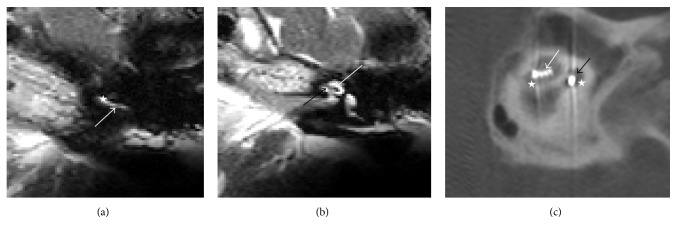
(a) MRI scalar pattern of a changing scala position (ST > SV) in Patient 1's basal turn. The arrow indicates the array's diminishing signal in the scala tympani. The star indicates the scala vestibuli in the basal turn. (b) MRI scalar pattern of a changing scala position (ST > SV) in Patient 1's second turn. The white arrow indicates the electrode's diminishing signal in the second turn. The black arrow indicates the scala tympani in the second turn. (c) FPT pattern of a changing scala position in Patient 1. The stars indicate the scala tympani in the basal and second turns. The white arrow indicates the scala vestibuli's position in the second turn. The black arrow indicates the scala tympani's position in the basal turn.
